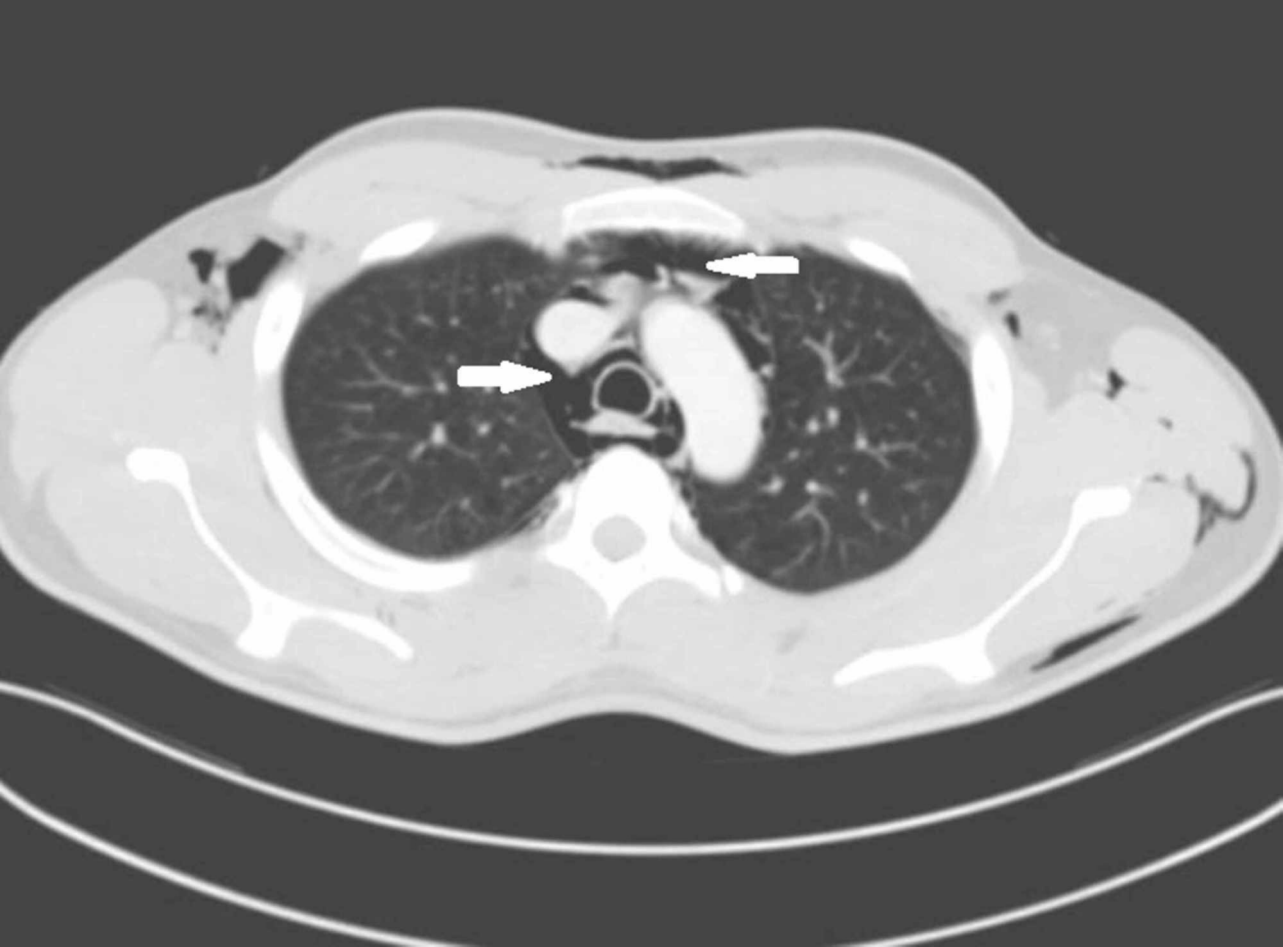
Contrast-enhanced computed tomography (CECT) of the chest showing pneumomediastinum (arrows)
